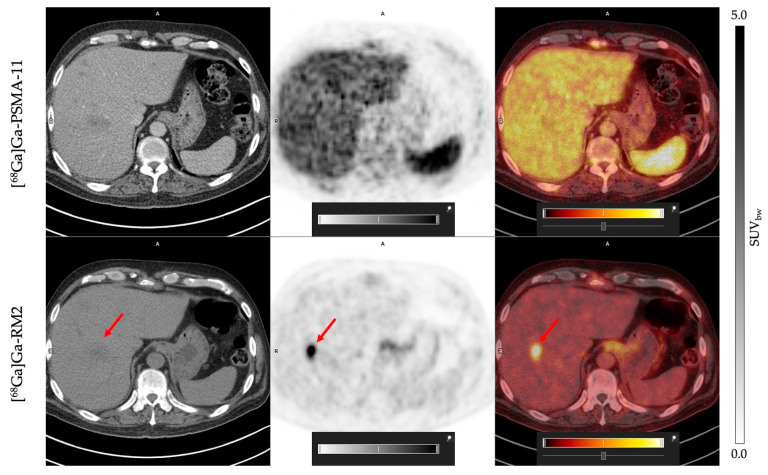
Patient 13: PET/CT [^68^Ga]Ga-PSMA-11 (upper row) and [^68^Ga]Ga-RM2 (lower row). Red arrows indicates a liver metastasis visible with [^68^Ga]Ga-RM2 and not detected with [^68^Ga]Ga-PSMA-11.
